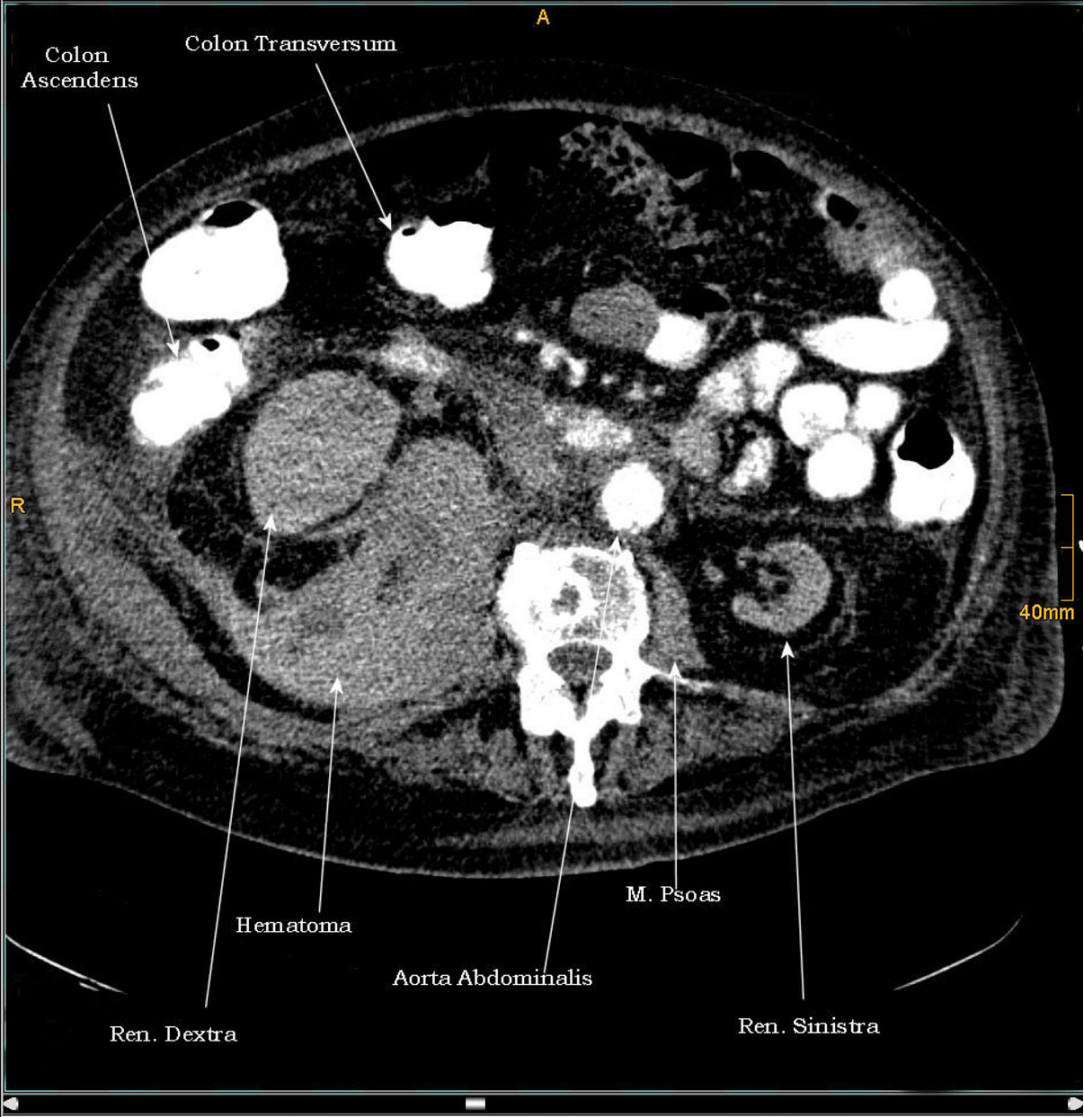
4^th ^case. CT - axial plan of the hematoma.
